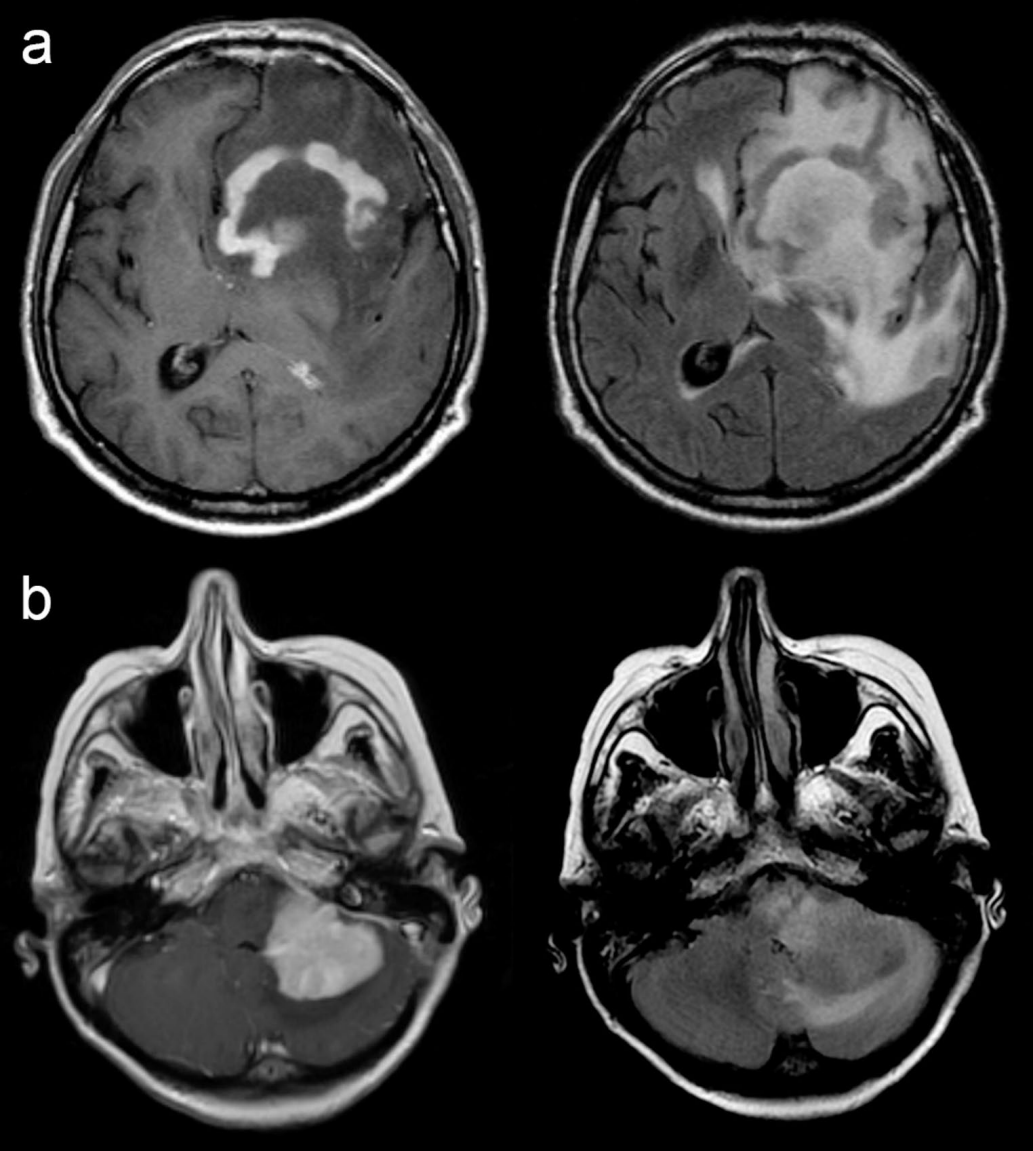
The difference in proportion of edema is depicted in the C + T1W and FLAIR images for a case of PCNSL in the upper row (a) and a case of SCNSL in the lower row (b). It is noteworthy that there is extensive edema in the PCNSL case, whereas the edema volume is comparatively less in the SCNSL case
